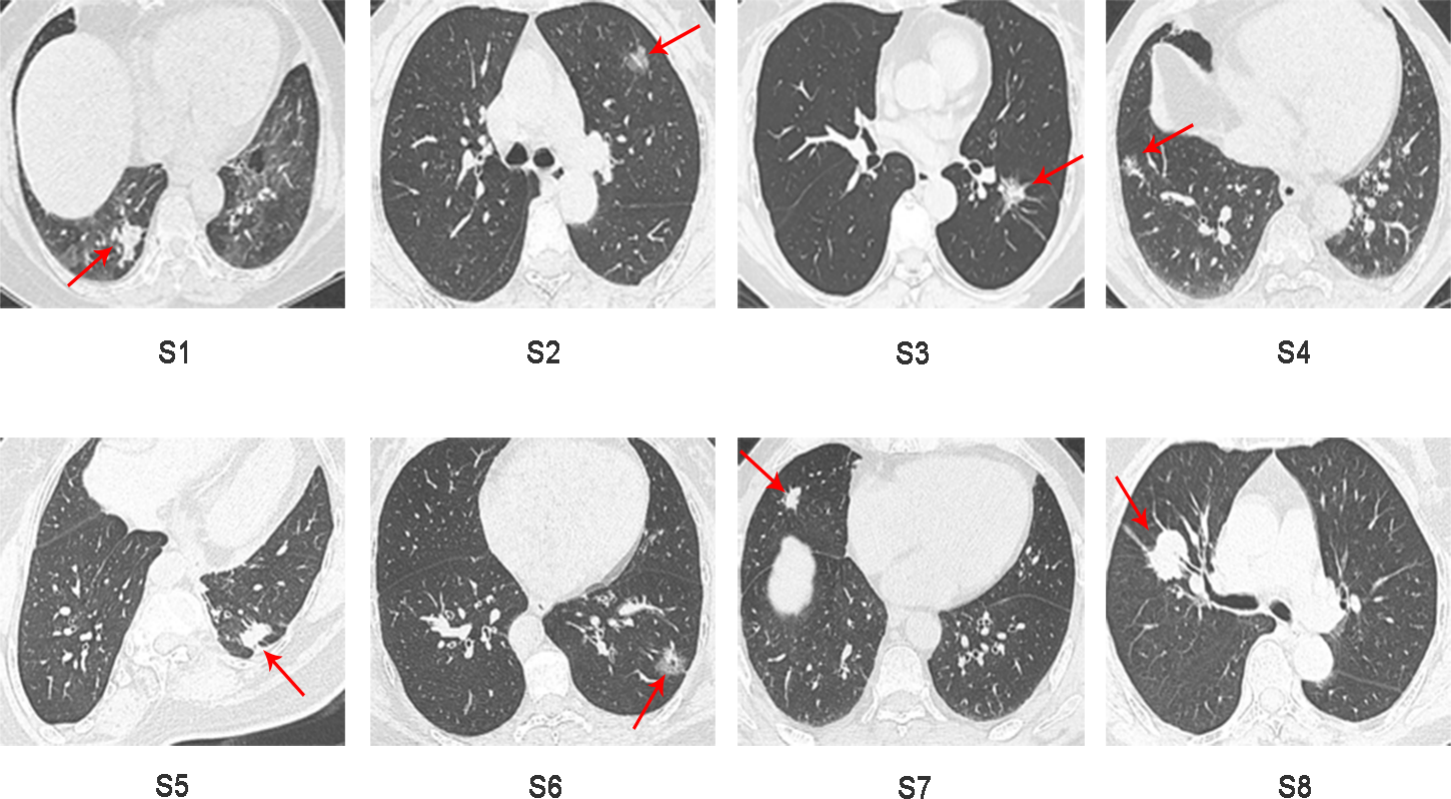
Preoperative CT imaging of the chest in eight patients with independent primary lung adenocarcinoma.
